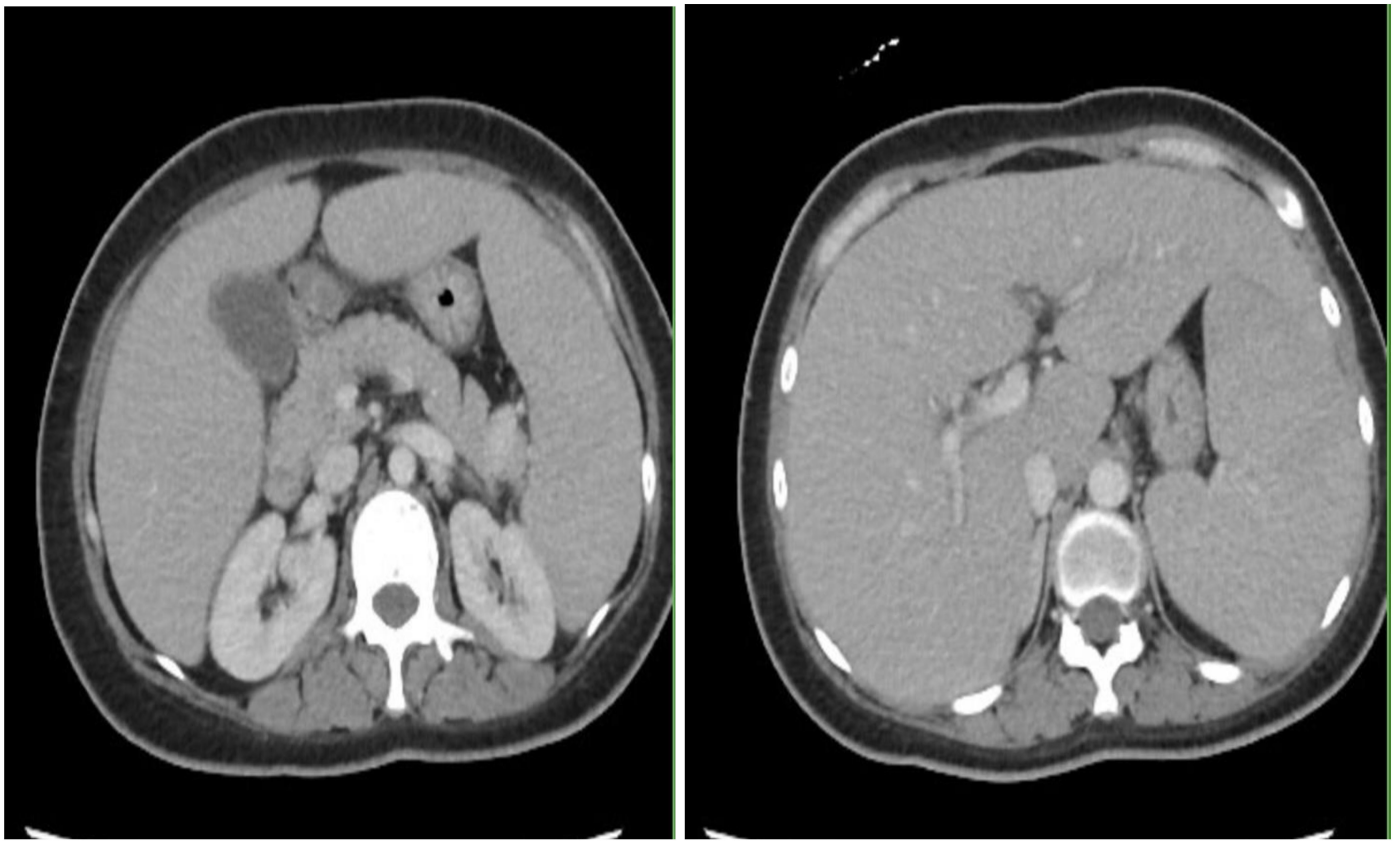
CT scan with contrast showing marked hepatosplenomegaly.
